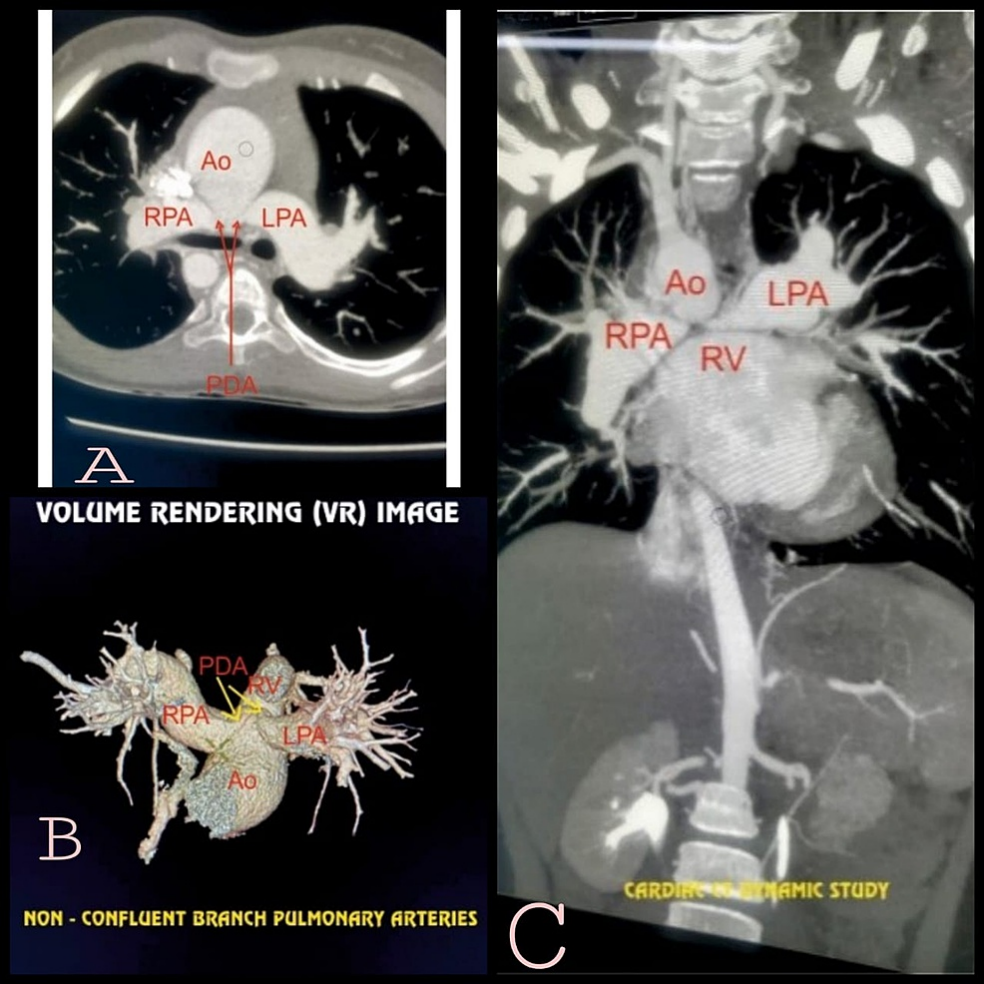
Volume rendering CT cardiac dynamic study showing non-confluent branch pulmonary arteries. Image credits: Dr. Viral B. Patel.
